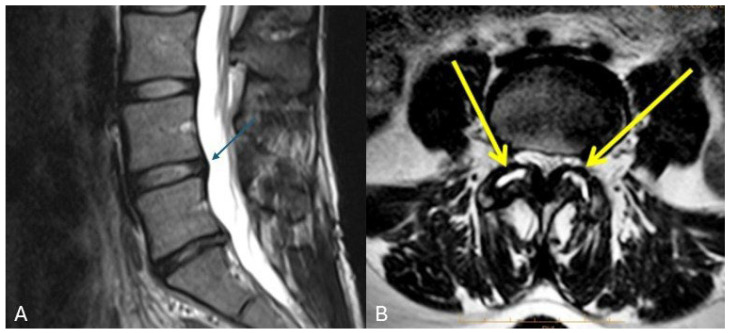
(A) MRI of a patient with discogenic low back pain. Note the L5-S1 discal herniation (blue arrow) associated with the discal degeneration as exemplified by the reduced signal on the T2-weighted imaging; (B) another patient’s MRI reporting a marked facet arthritis (yellow arrows).
